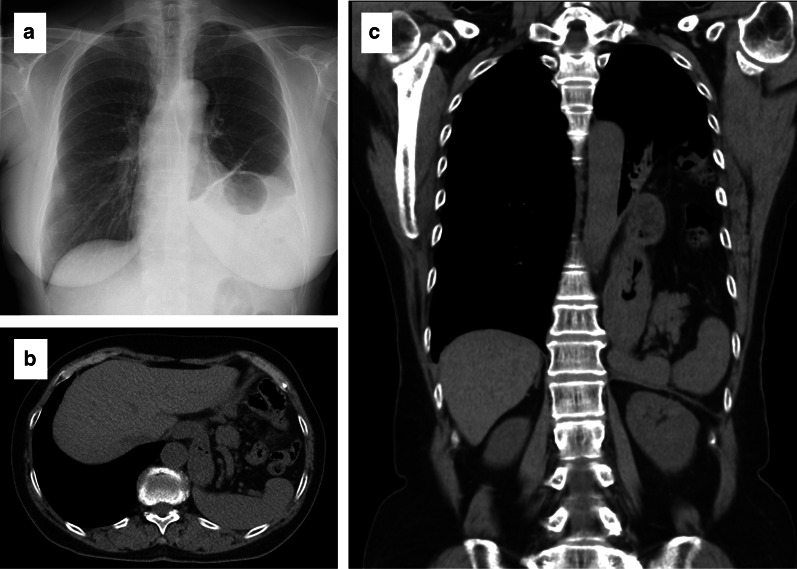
a Preoperative images of the Bochdalek hernia. The chest radiography on admission shows a lesion containing bowel gas in the left thoracic cavity. b Axial view images of the CT scan showing prolapse of the stomach, small intestine, colon, pancreas, and spleen into the left thoracic cavity. c Coronal view images of the CT scan showing same as above
